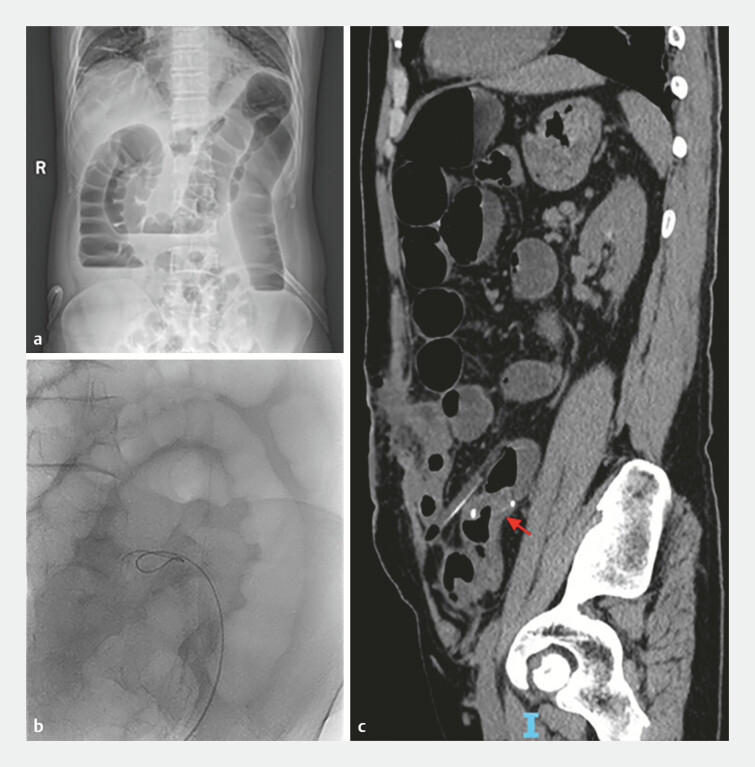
Evaluation prior to endoscopic ultrasound‑guided stricturotomy.
